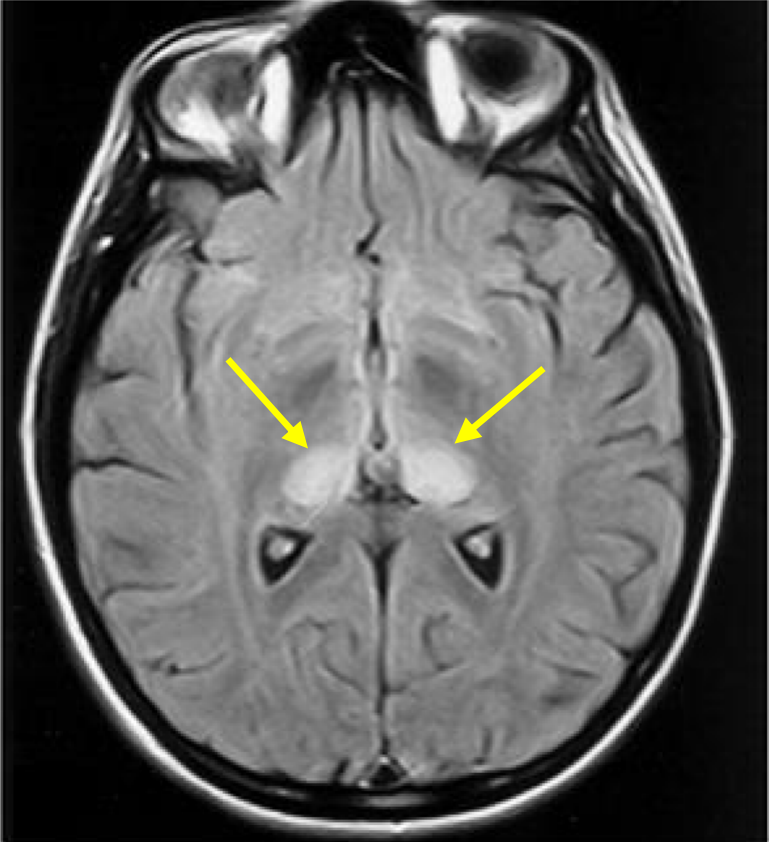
MRI brain in variant CJD - axial FLAIR section at the level of the basal ganglia demonstrating bilateral hyperintensity in the pulvinar nuclei of the posterior thalamus (arrows)
